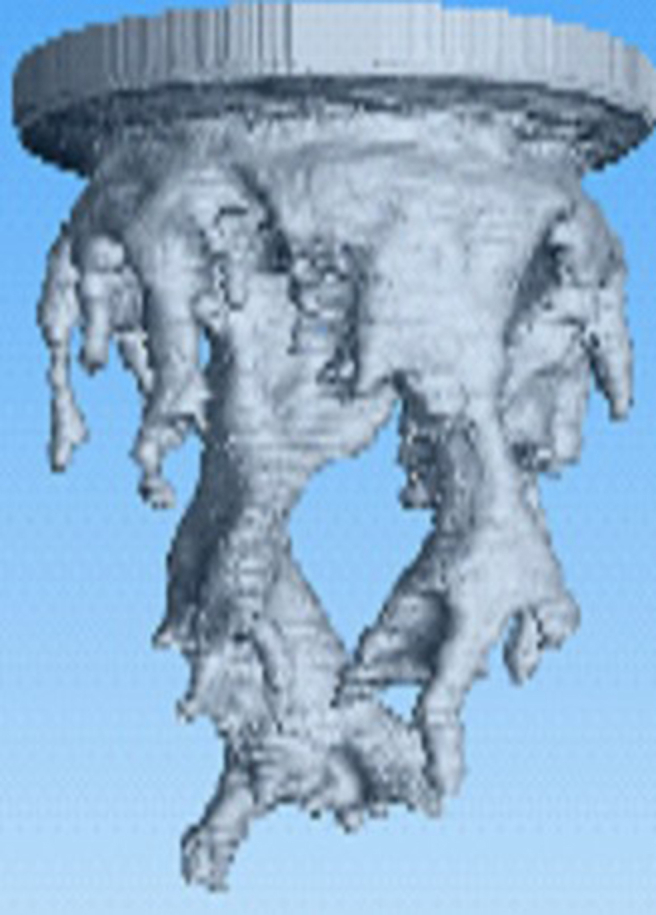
Experimental results of *3D* VF in a cylindrical packed bed. The results were obtained with a micro-focused x-ray tomography scanner. Image adapted from Suekane [22].
